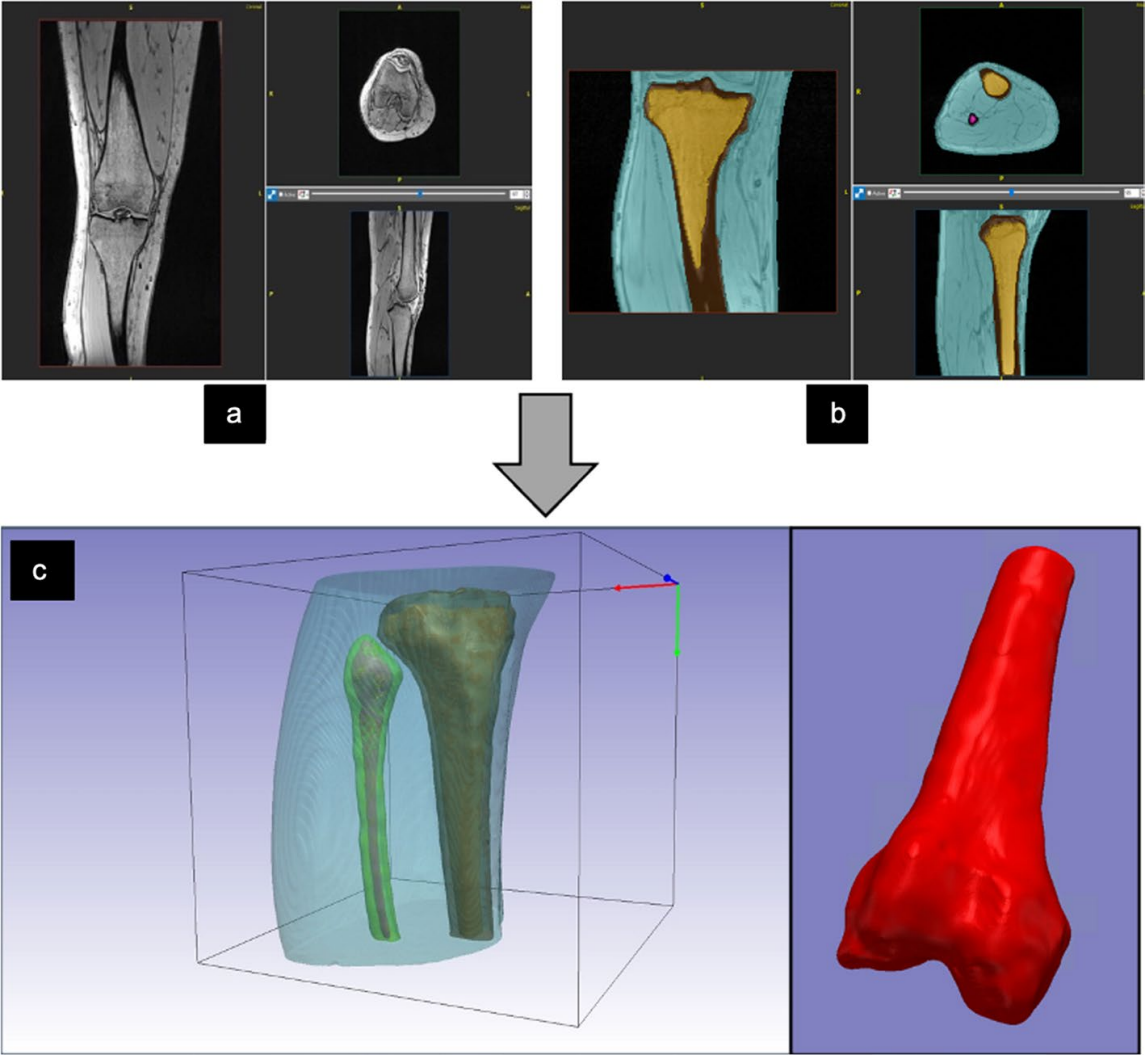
a Coronal, sagittal, and axial, view of knee MRI scan of one of the participants. b Segmented cortical and trabecular bone of knee joint MRI Images. c the exported 3D STL model of the segmented cortical, trabecular bone of the knee joint
